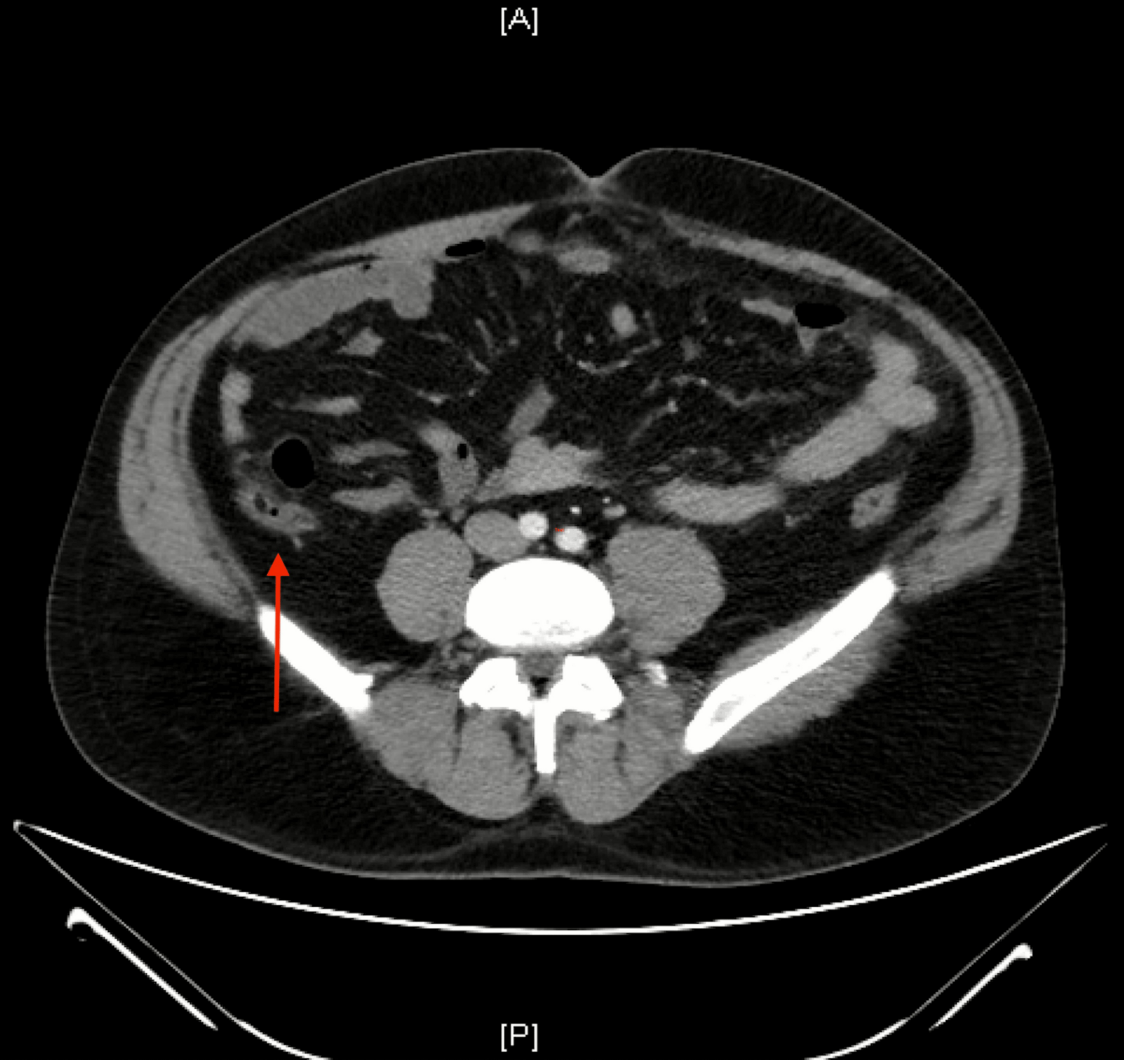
Axial CT scan of the lower abdomen The red arrow shows the enlarged appendix, which is distended by fluid. A: anterior; P: posterior
